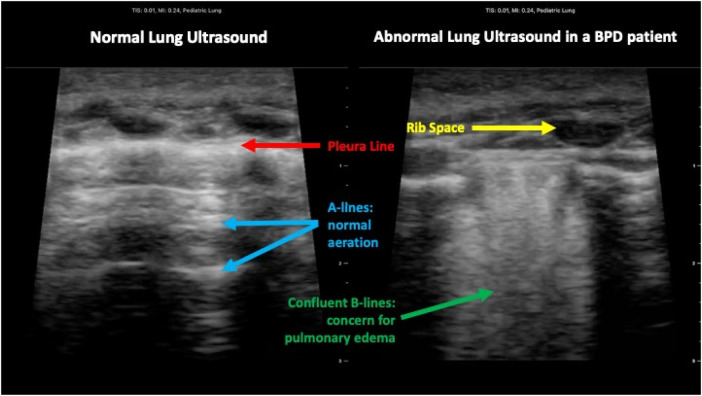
Comparison of normal lung ultrasound to lung ultrasound in BPD.
